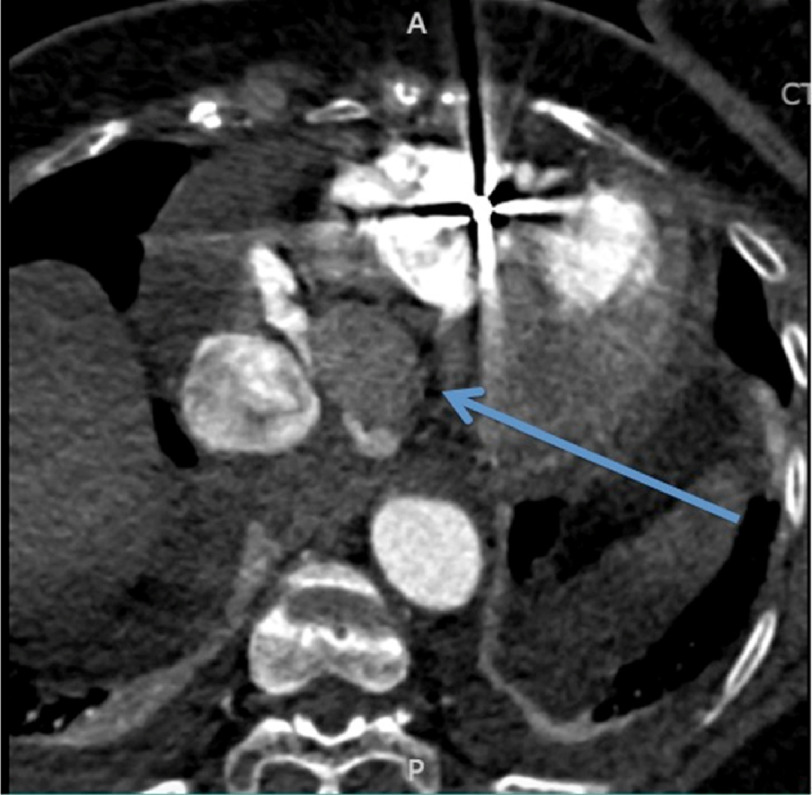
Axial slice showing a magnified view of the heart from a CTA of the chest with contrast, demonstrating (as indicated by the arrow) the mass with imaging features most suggestive of a cystic tumor of the atrioventicular (AV) node.
